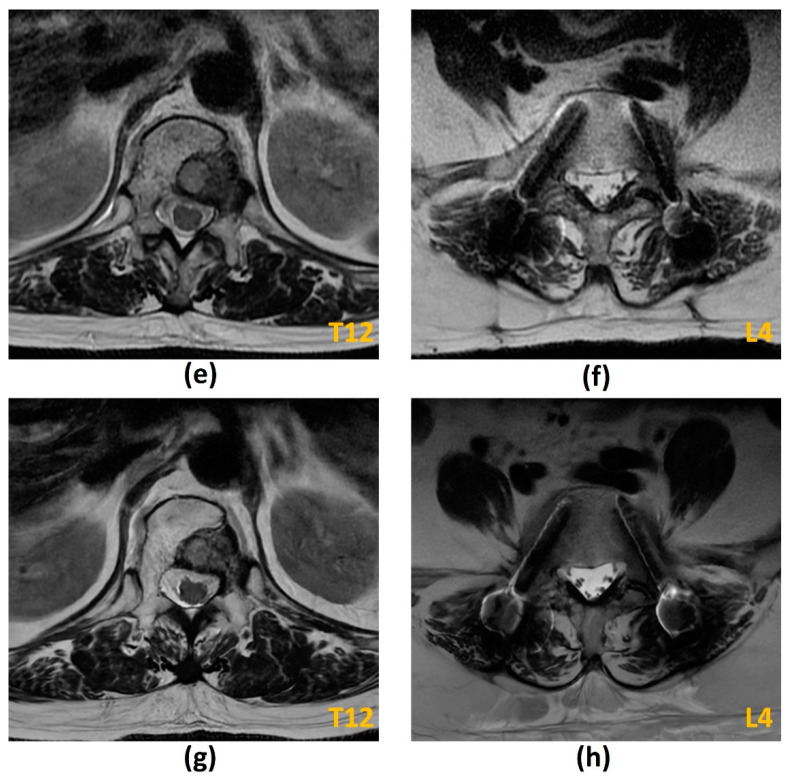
Spinal instrumentation with titanium screws in a patient with spinal metastasis. Standard sagittal STIR (a) and post-contrast fat-suppressed T1-weighted (b), and axial T2-weighted images at the level of T12 (e) and L4 (f). Six-month follow-up MRI was performed using AIR^TM^ Recon DL with sagittal STIR (c) and post-contrast fat-suppressed T1-weighted (d), and axial T2-weighted images at the level of T12 (g) and L4 (h). AIR Recon DL improves resolution and soft tissue contrast, significantly improving the diagnostic quality of images while reducing scan times.
